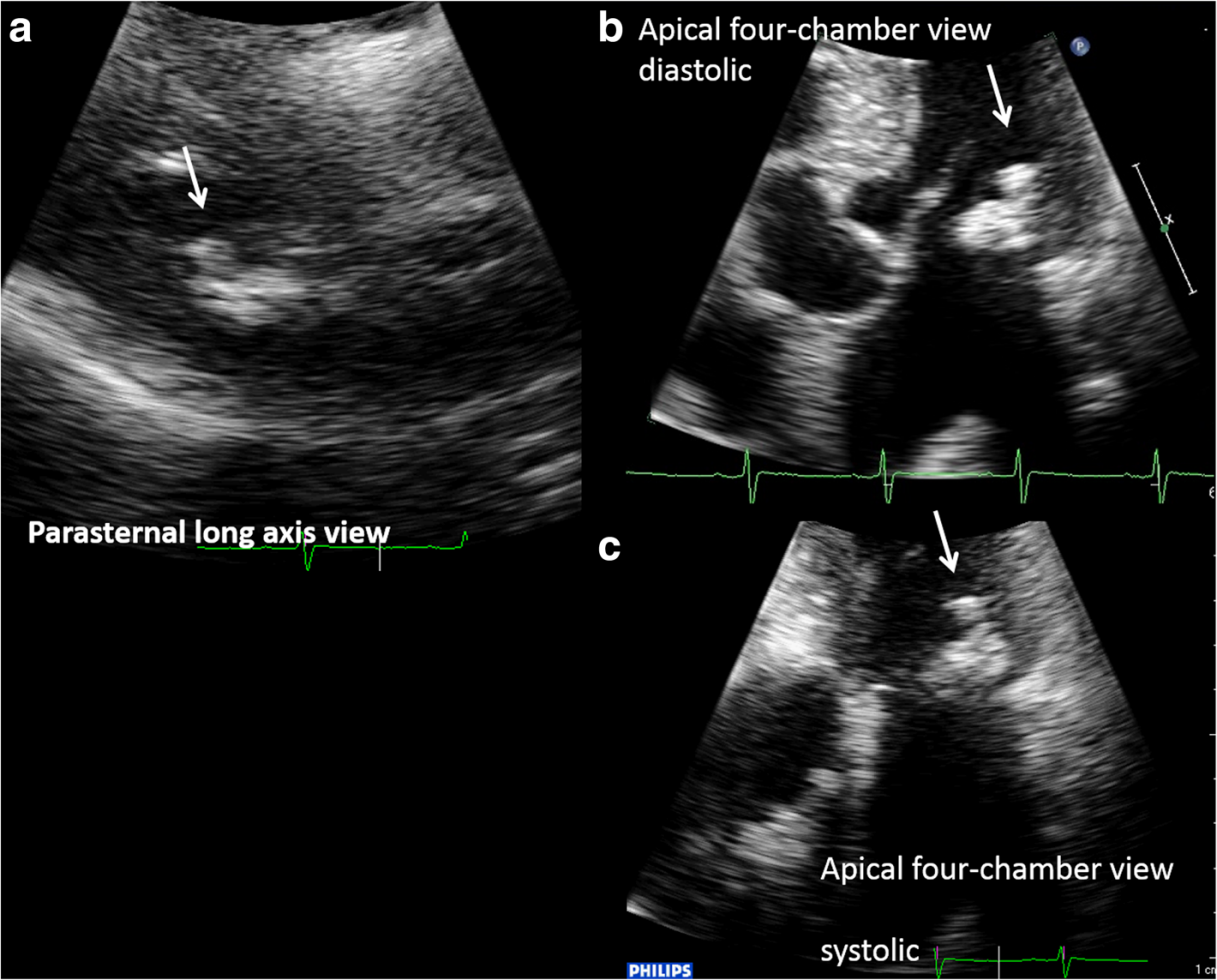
Transthoracic echocardiography. a Parasternal long axis view. b Apical four-chamber view (diastolic). c Apical four-chamber view (systolic). Transthoracic echocardiography revealed a highly mobile echogenic mass (*white arrow*), 8 × 6 mm, attached on the ventricular side of mitral annulus, with severe calcification
